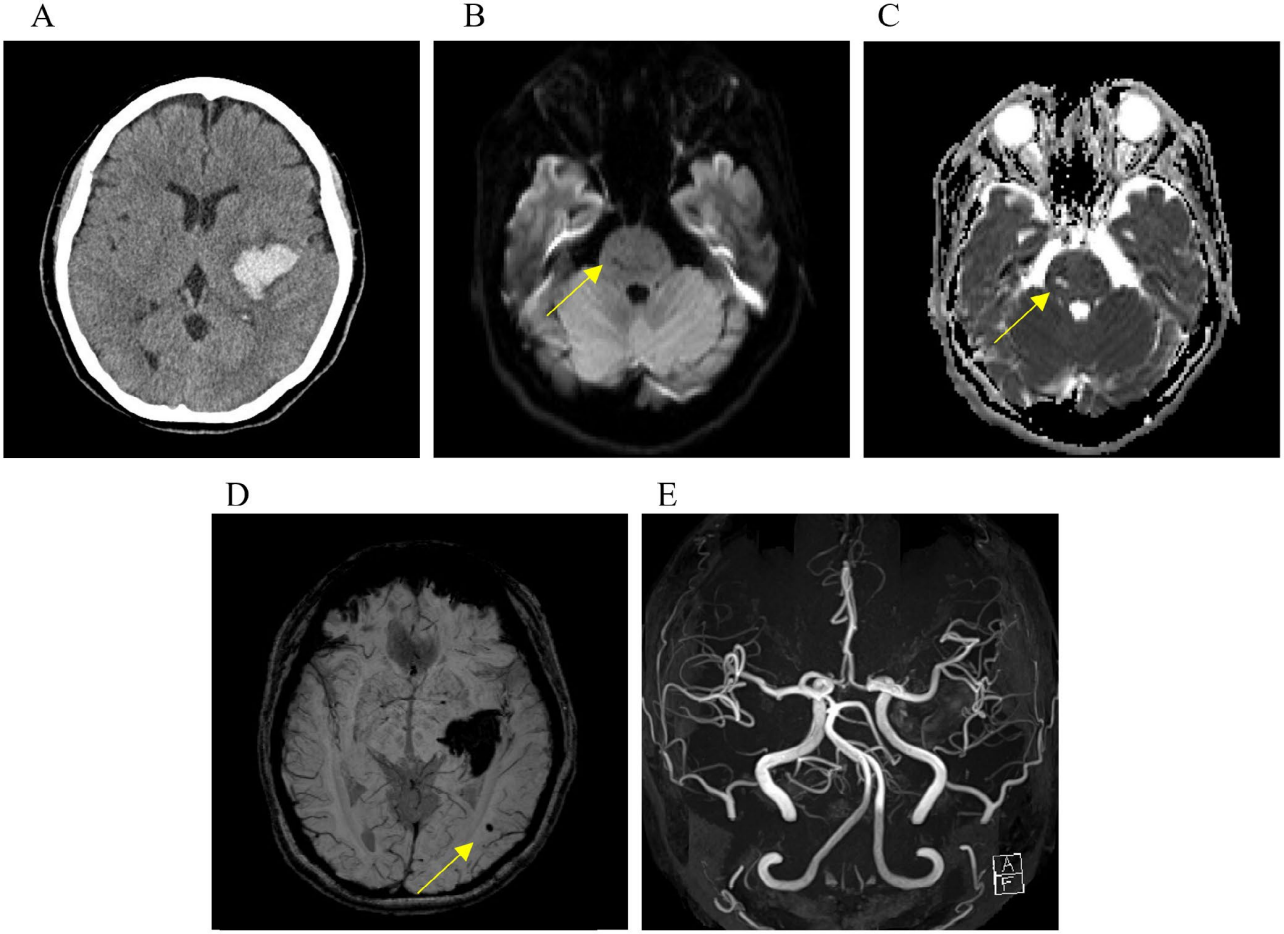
Computed tomography (A) showing high‐signal lesions in the left putaminal region. Diffusion‐weighted (B) and apparent diffusion coefficient magnetic resonance images (C) revealing an old small brainstem infarction. Susceptibility‐weighted imaging (D) showing microbleeds. No stenosis or irregularities of large cerebral arteries were observed on magnetic resonance angiography (E).
